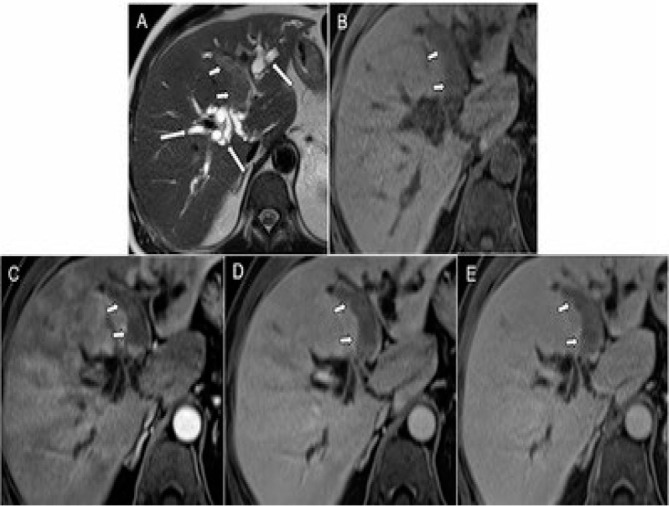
Axial MRI slices in axial T2SE sequence (A), T1GRE with pre-contrast fat saturation (B), and post-contrast in arterial phase (C), portal (D), and late 5 min (E) where an endoluminal mass (short white arrows) is observed hypointense on T1, with intermediate-high signal on T2, and hypovascular impregnation, which occupies and dilates the lumen of the medial segmental biliary branch. Note the dilation of the intrahepatic bile duct in the other segments (long white arrows).
